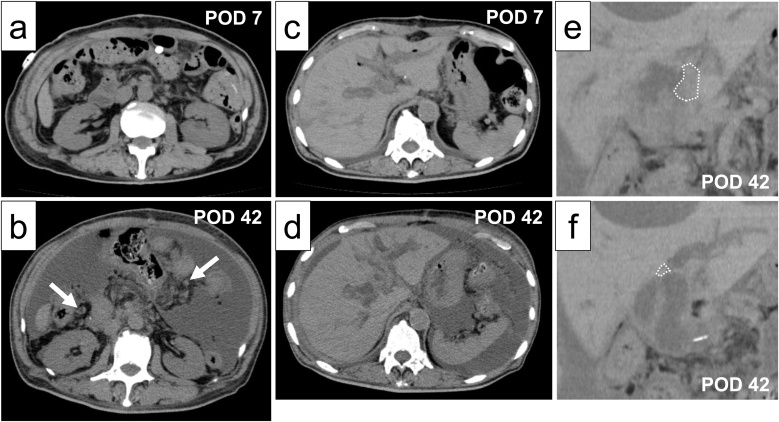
Abdominal CT scan showing multiple peritoneal nodules (arrow) with a large amount of ascites at POD42 (b) compared to POD 7 (a) and dilation of the intrahepatic bile duct at POD 42 (d) compared to POD 7(c). Local recurrence at the site of the cholejejunostomy is shown (e, f). POD: postoperative day.
